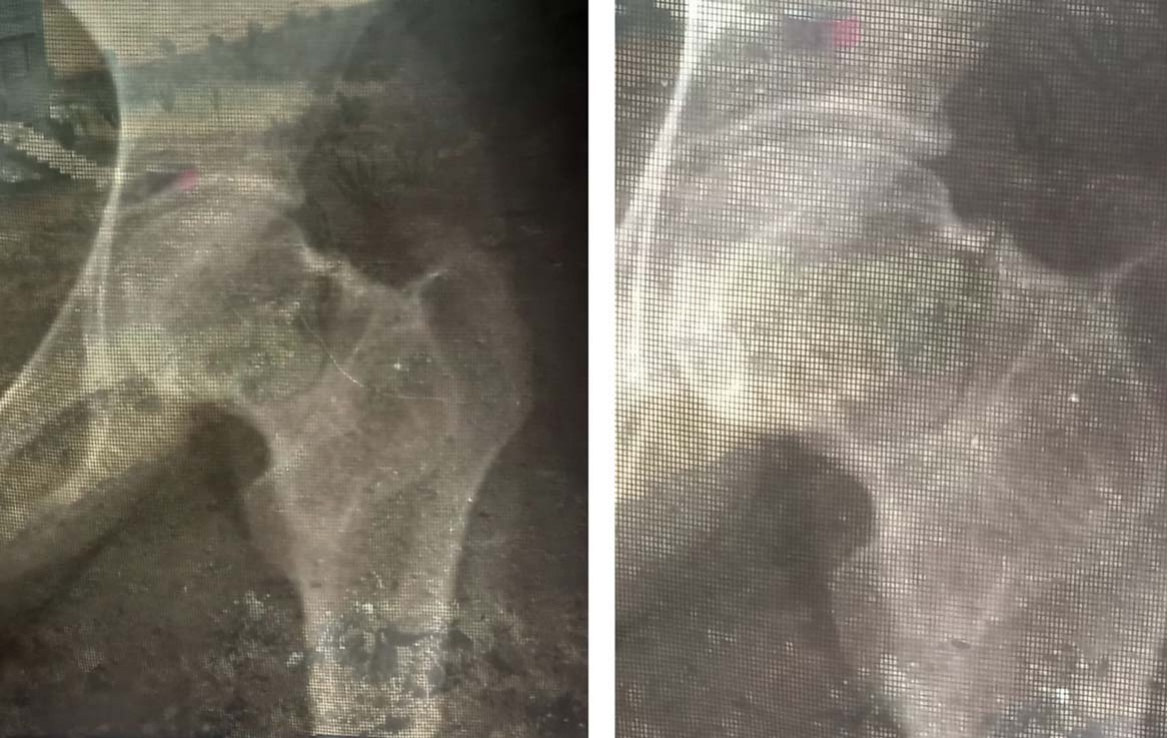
AP X-ray showing intracapsular neck of femur fracture with underlying lesion.
